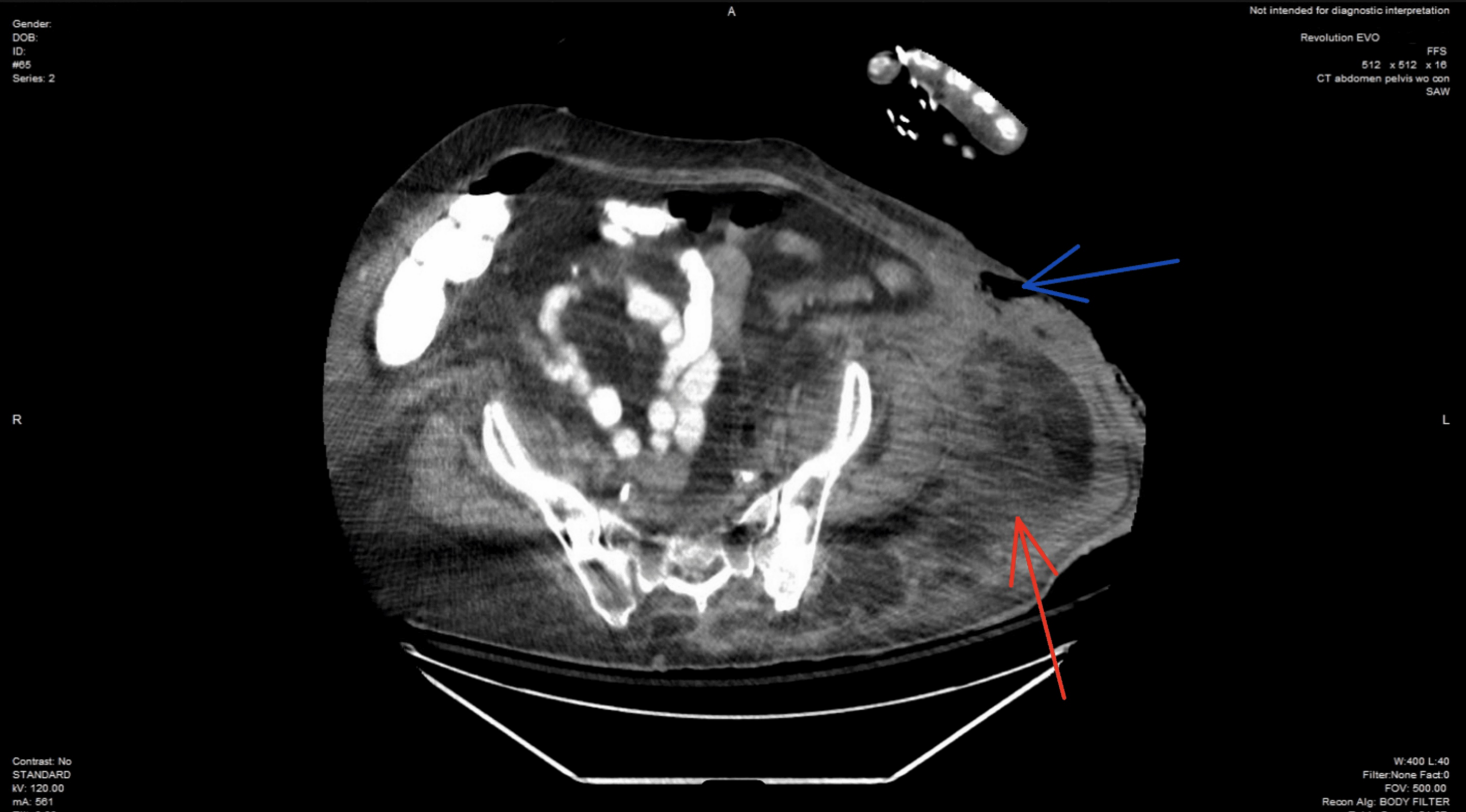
Axial view at the level of the sacrum, CT scan of the abdomen/pelvis shows subcutaneous emphysema (blue arrow), and subcutaneous fat standing (red arrow) CT: computed tomography
